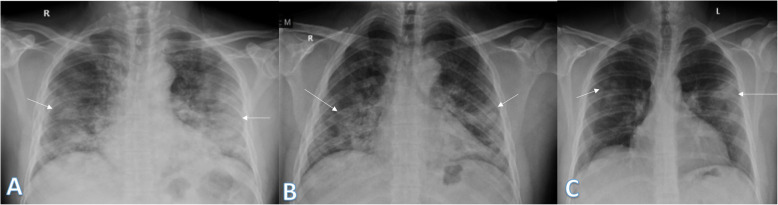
Three different patients with positive COVID-19. a 54-year male was complaining of SOB, fever, and cough. Initial chest X-ray showed bilateral mid and lower zonal air space consolidation opacities more on the left side with neither perihilar nor peripheral distribution (arrows). The severity score was 2 for the right lung and 3 for the left lung, so TSS was 5. b 36-year male was complaining of fever. Initial chest X-ray showed right perihilar (long arrow) and left neither peripheral nor perihilar air space consolidation opacity (short arrow) of the mid and lower lung zone. The severity score was 2 for each lung, so the total severity score was 4. c 27-year male was complaining of cough and fever. Initial chest X-ray showed right small midzonal pulmonary nodules (short arrow), left midzonal neither peripheral nor perihilar air space consolidation opacities are seen (long arrows), and the severity score was 1 for each lung, so TSS was 2
